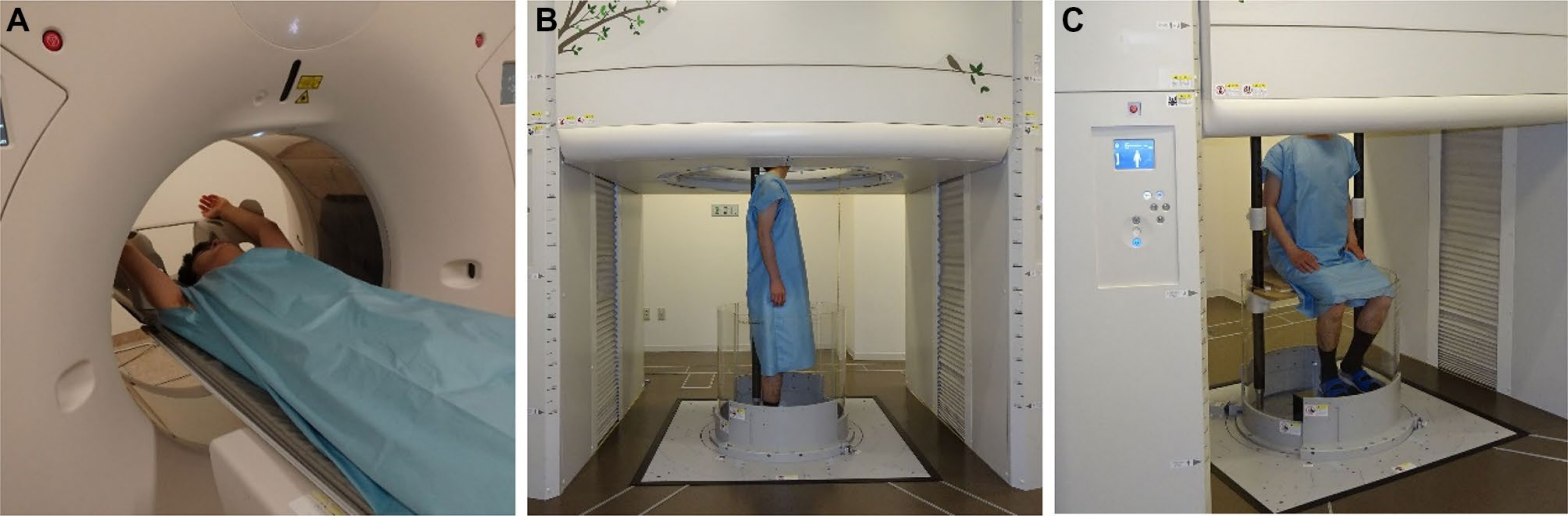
Conventional CT examination in the supine position (A), upright CT examination in the standing position (B) and upright CT examination in the sitting position (C). Conventional CT in the supine position (A) was performed with the subject’s arms raised during both deep-inspiration breath-hold and expiration breath-hold. Upright CT examinations in the standing position (B) and sitting position (C) were performed with the subject’s arms down during both deep-inspiration breath-hold and expiration breath-hold. For safety during upright CT scanning, an acrylic wall encircling the body was added to prevent falls, with a pinch prevention mechanism and contact interlock control mechanism. Furthermore, to stabilize patients while standing, a back-support pole, 2.3-m long and made of carbon, was included; it was mounted between the floor and the top of the system (B). The mounting position could be adjusted based on the subject's bodily dimensions or the scan conditions. To support patients who are frail or elderly, a Velcro band can be attached to the pole and loosely wrapped around a patient's body. In this study, the subjects were healthy volunteers; thus, we did not use the Velcro band.
